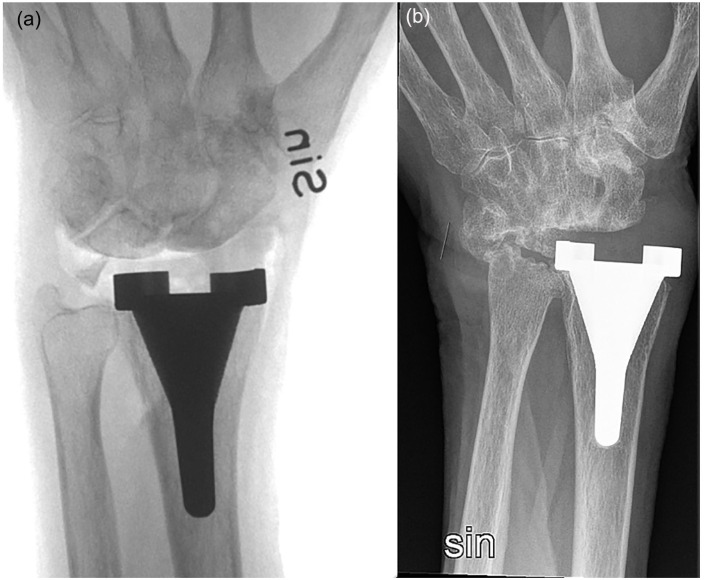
Anteroposterior radiographs (a) preoperatively and (b) 1 year postoperatively.
